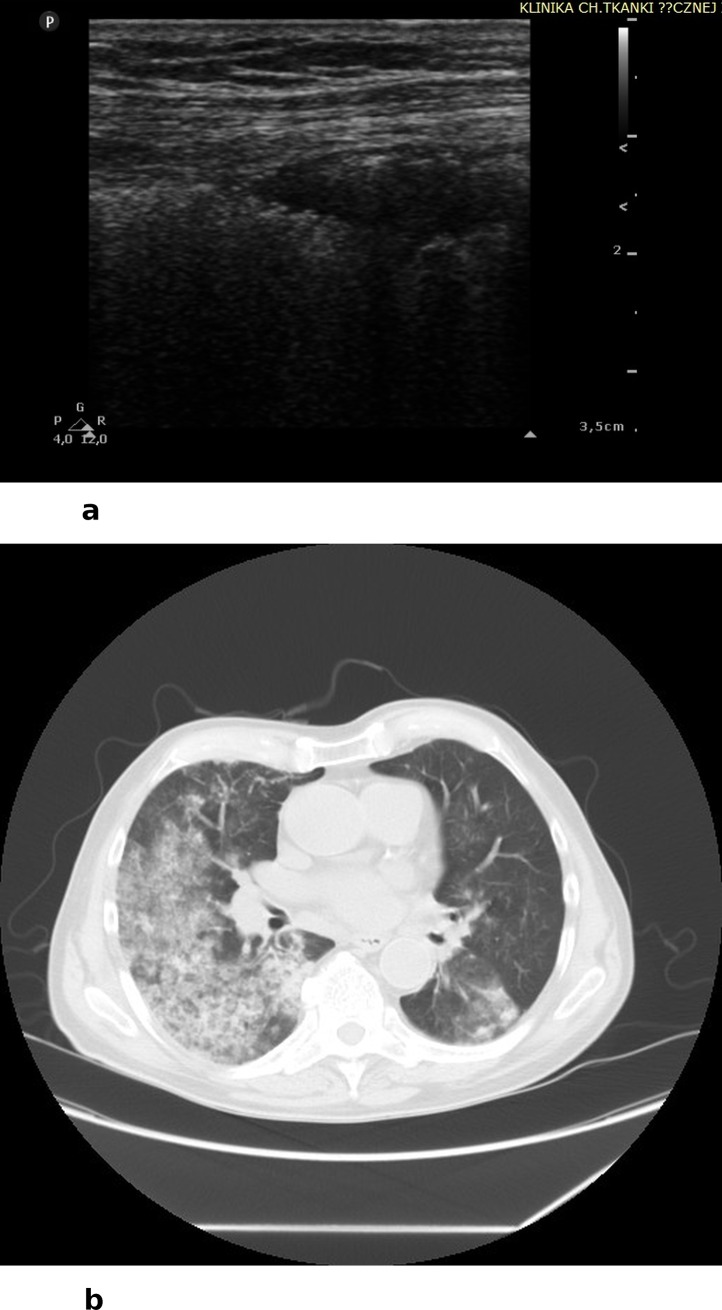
A) LUS: oval, hypoechoic subpleural consolidation in DAH, B) CT: Features of alveolar hemorrhage secondary to vasculitis.
